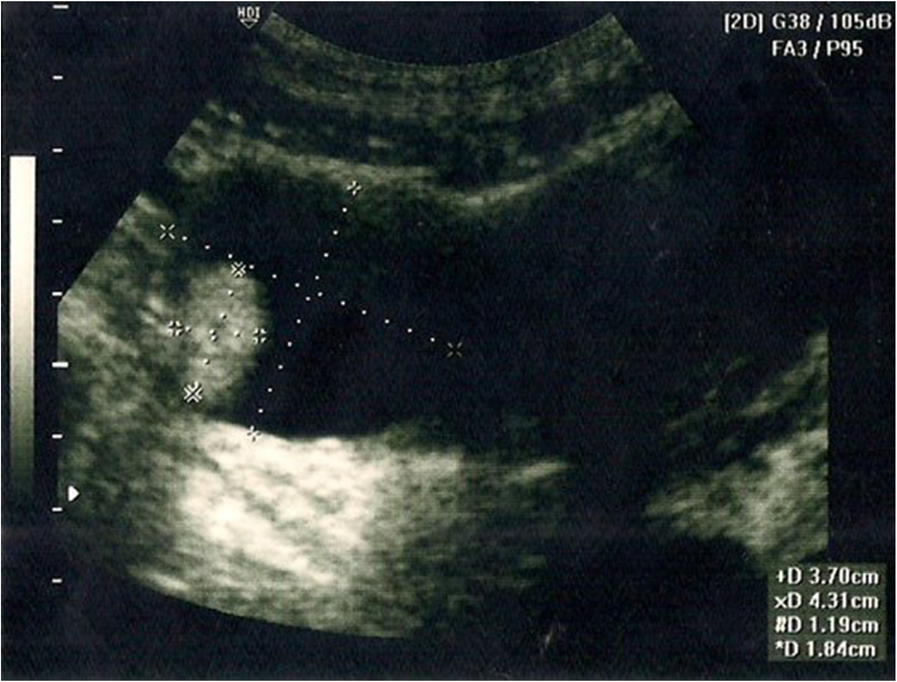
Transabdominal ultrasound revealed a well-defined, hyperechoic mass (4.3 × 3.7 cm) with an appearance resembling that of a teratoma, just adjacent to the uterus.
